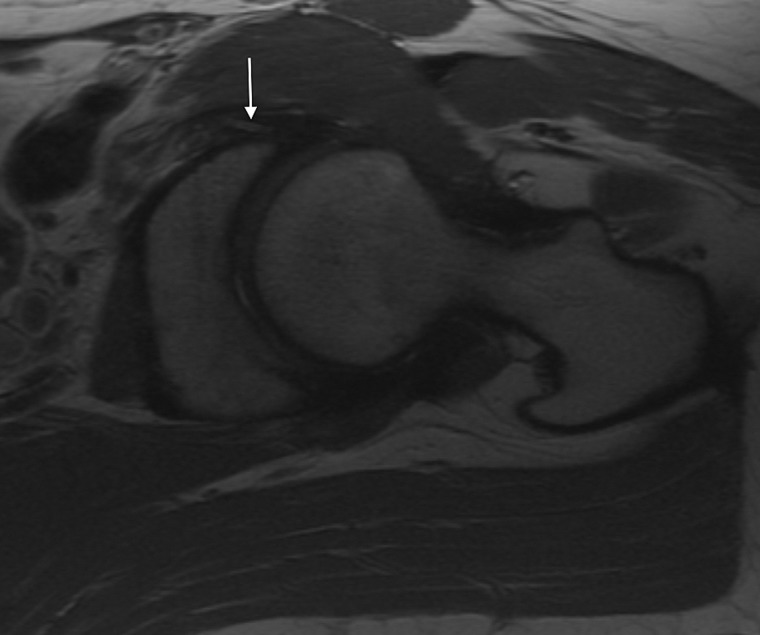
Axial MRI demonstrating medial cortical perforation (arrow) 77 × 64 mm.
